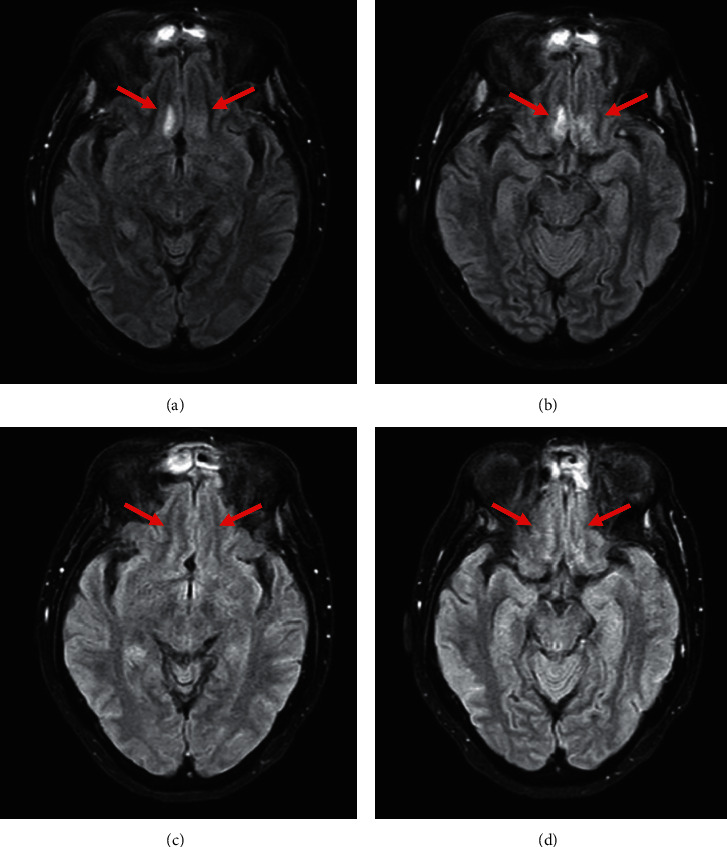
(a, b) FLAIR sequence showed hyperintense signal in cortical anterobasal frontal lobes (arrows) at the beginning of the treatment (first week). (c, d) FLAIR sequence showed reduced hyperintense signal in cortical anterobasal frontal lobes (arrows) at 5 months follow-up.
